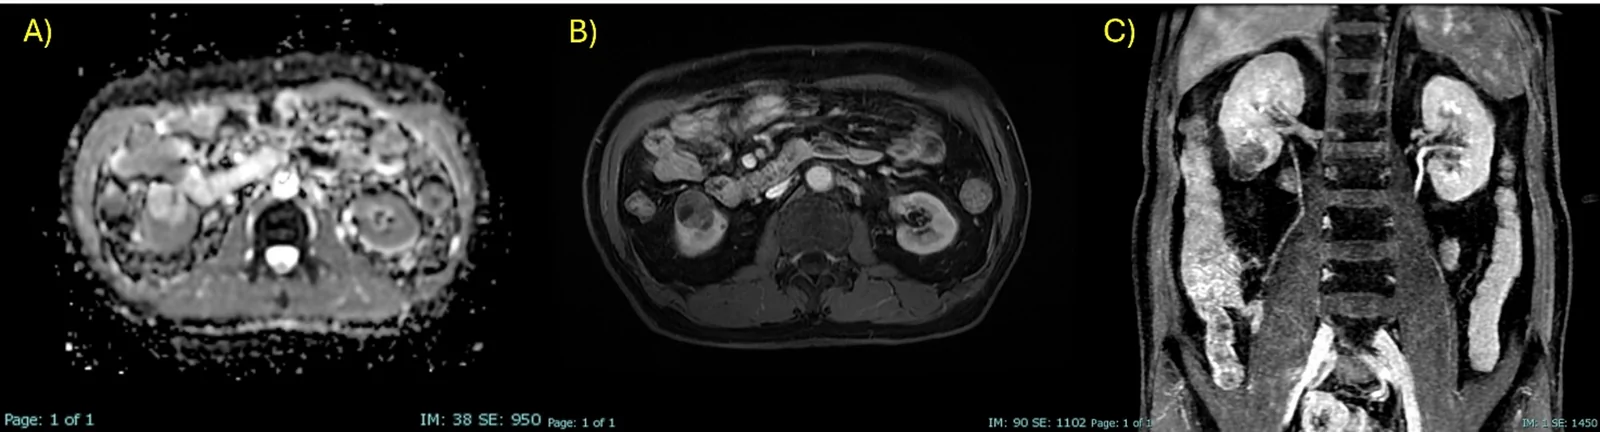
MRI images: axial apparent diffusion coefficient (ADC) map (A) and contrast-enhanced T1-weighted sequences acquired on axial (B) and coronal (C) planes showed indeterminate signal characteristics of the mural component
